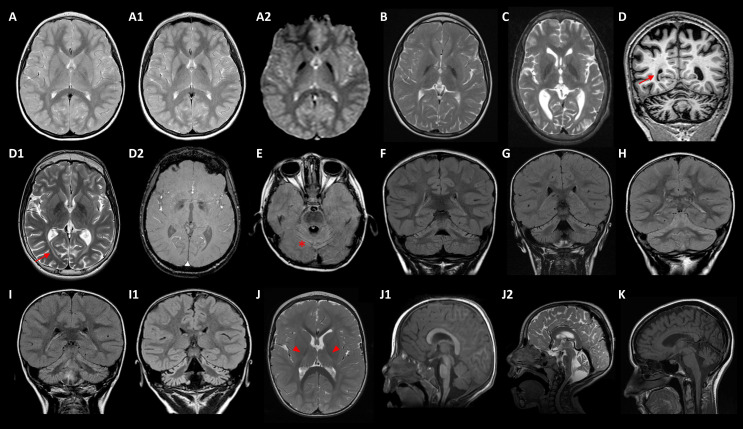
Main neuroimaging features. (A–A2) MD-020/*GLB1*: axial TSE (turbo spin echo) T2 weighted image at ages 6 (A) and 11 (A1) showing progression of *globus pallidus* (GP) hypointensities, demonstrating iron deposition in axial T2* GRE (gradient echo) image (A2) at 11 years old. (B) MD-137/*FUCA1* and (C) MD-153/*TPP1* revealing T2-weighted images (T2WI) GP hypointensities at ages 10 and 14, respectively. (D–D2) MD-189/*KIF1A* at 17 years old: coronal T1-weighted image showing cerebral and cerebellar atrophy (D), axial T2WI revealing GP hypointensity (D1) and SWI, susceptibility weighted imaging (D2) showing iron deposition; the patient also presented periventricular and deep white matter abnormal signal (arrows). (E) MD-200/*KIF1A* at age of 4: axial FLAIR (fluid attenuated inversion recovery) image revealing global cerebellar atrophy with cortical hyperintensity (* tram-track sign). (F–H) Coronal FLAIR images uncover predominantly upper cerebellar cortical hyperintensity in patients MD-106 at age of 3 (F), MD-041 of 4 (G), and MD-320 of 4 (H), with *ITPR1* variants. (I–I1) MD-323/*PMPCA*: Coronal FLAIR images at 2 years old (I), and at 8 years old (I1) demonstrating progression of cerebellar atrophy with cortical hyperintensities. (J–J2) MD-181/*PLEKHG2* from 2 (J,J1) to 5 years old (J2): T2WI (J) showing thalamic and posterior internal capsule hyperintensities (head arrows), and mild deep white matter hyperintensity; mid-sagittal T1 weighted image (J1) and midsagittal T2WI (J2) demonstrating progression of cerebellar atrophy. (K) MD-270/*HEXA*: mid-sagittal T2WI revealing cerebellar atrophy at disease onset (23 years old).
